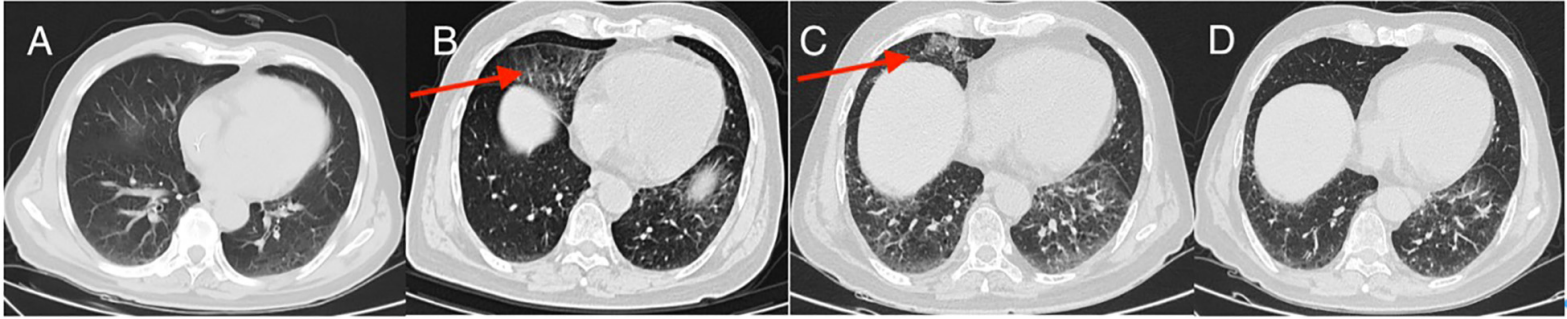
CT manifestations of pneumonia (A–D); (A) no signs of pneumonia after three cycles of immunotherapy; (B) immune pneumonia after 10 cycles of immunotherapy (red arrow); (C) immune pneumonia after 16 cycles of immunotherapy (red arrow); (D) immune pneumonia after 10 cycles of immunotherapy disappeared after 20 cycles of immunotherapy.
